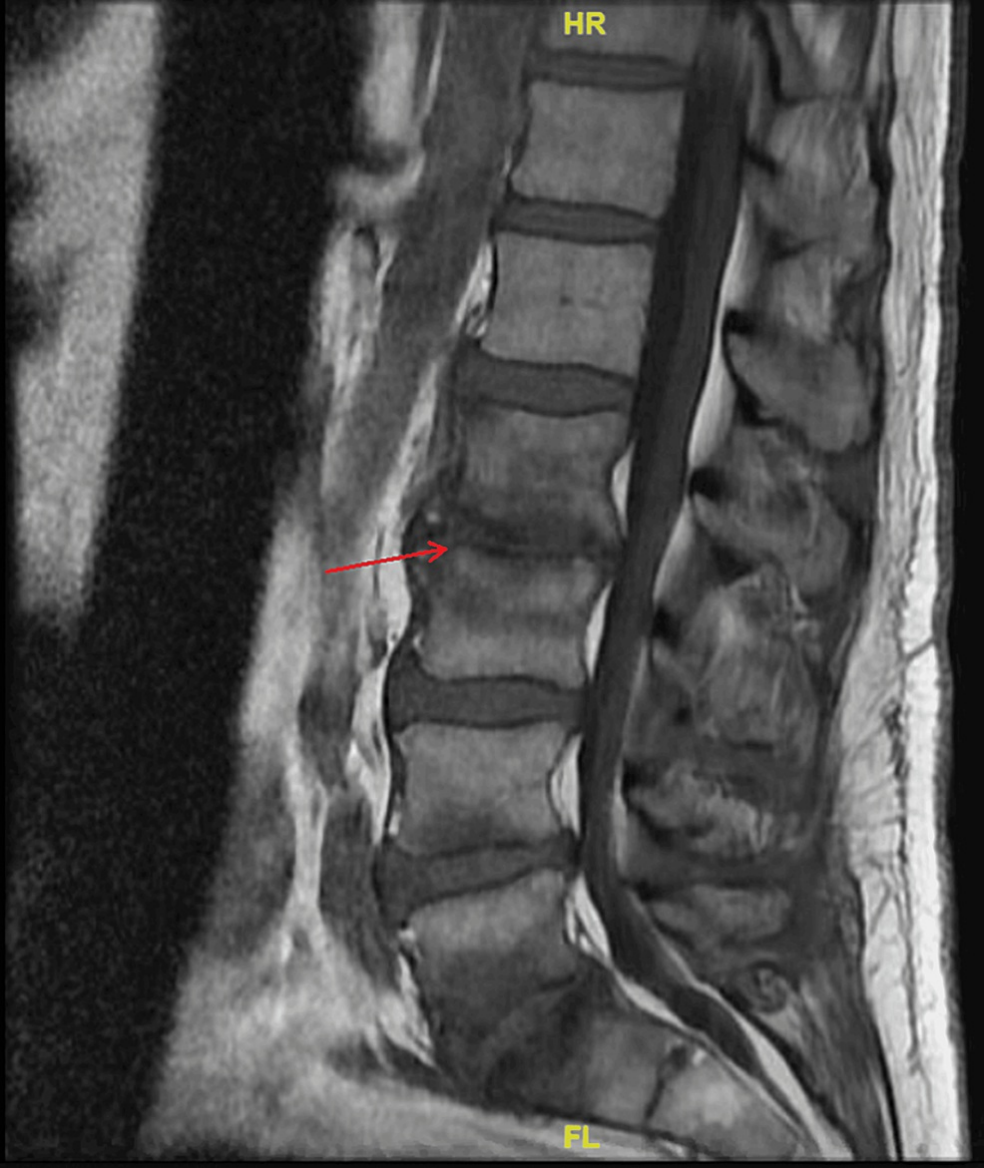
T1 sagittal MRI L-spine. The red arrow at the L4-L5 level demonstrates marked marrow signal changes suggestive of osteomyelitis/discitis.
